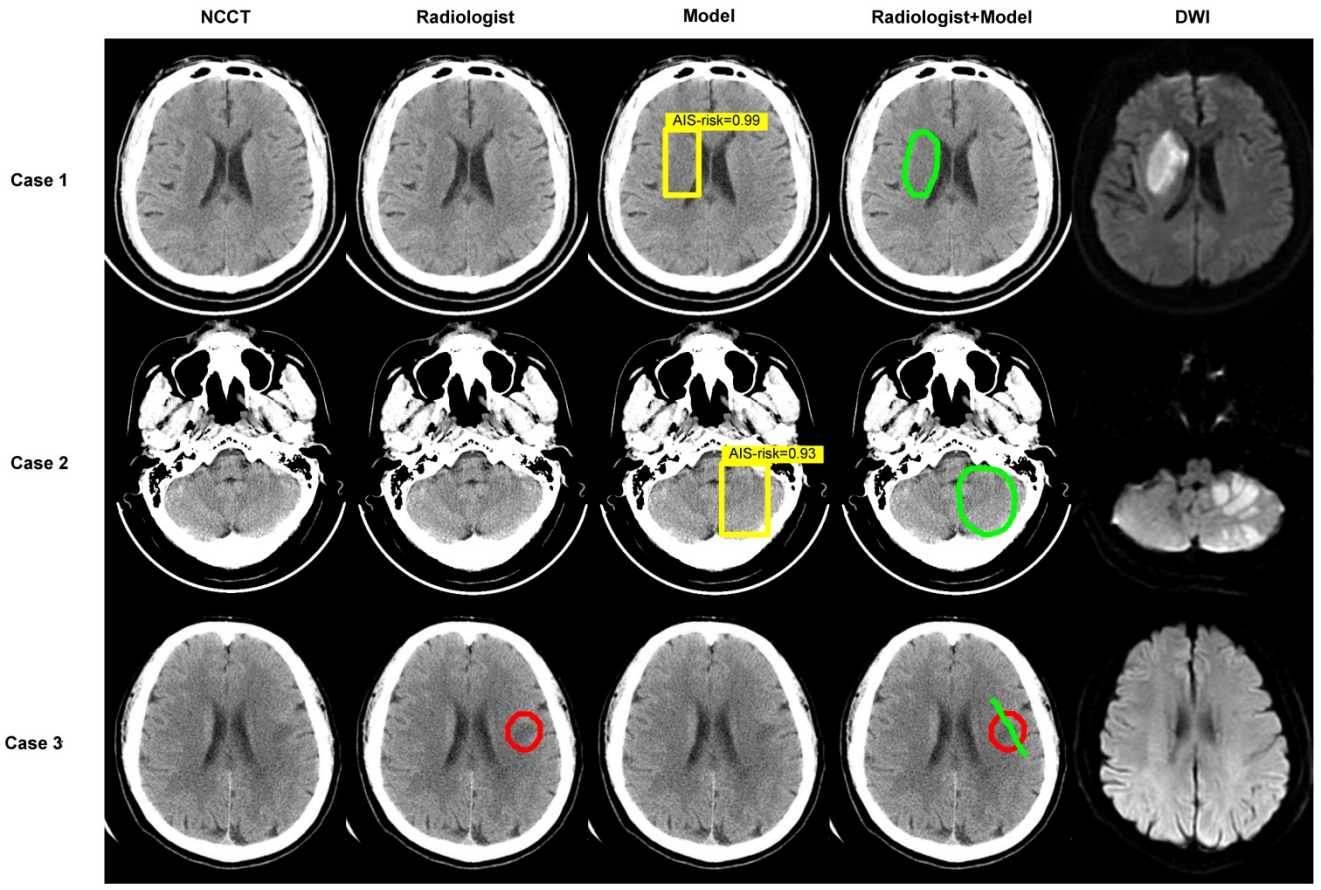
Examples. Images show the results of two experienced radiologists without and with the assistance of the deep-learning model for diagnosing. The first two cases are AIS patients and the last was imaging-negative patient. Within each case, left column shows a NCCT image, second column shows the result of radiologist read, middle column shows result of model diagnose (the yellow box represents the location of AIS and the probabilities calculated by the model in this region are 0.99, 0.93), fourth column shows the result of “radiologist + model” (the green circle or line), and right column shows corresponding DWI.
